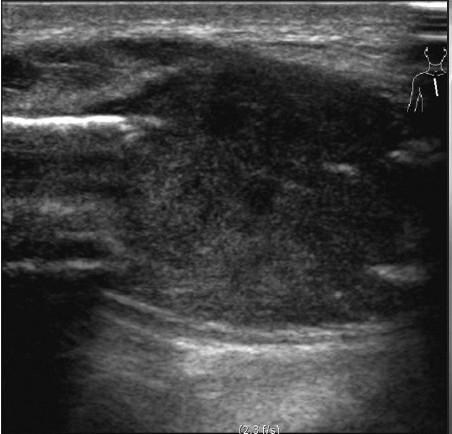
A 32-year-old male with concurrent sternal and pulmonary LCH. (a) Sagittal ultrasonogram of the sternum shows a heterogeneous low-echoic mass causing cortical disruption and soft-tissue extension.
